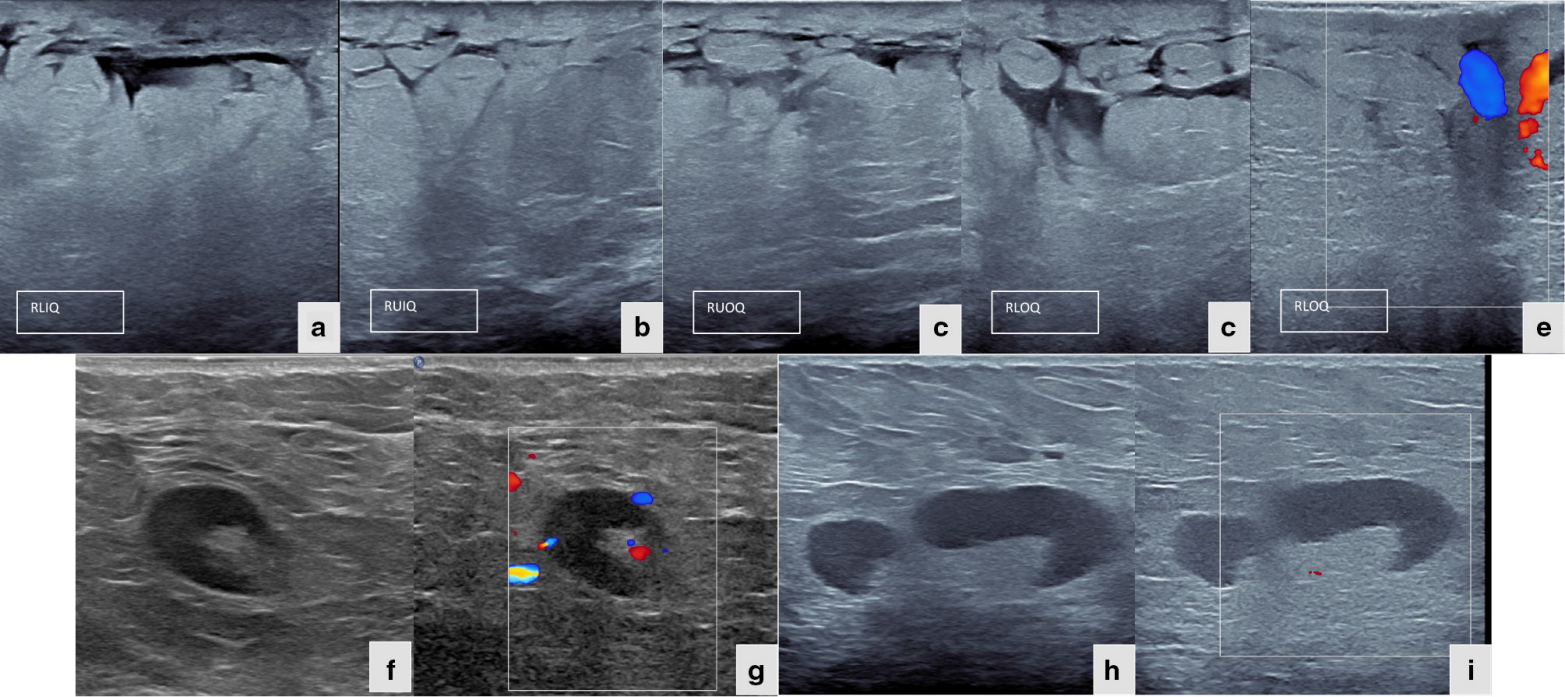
(a–e) Ultrasound and colour doppler of the four quadrants of the right breast shows skin thickening with oedematous changes involving the fat lobules of the breast and mildly increase blood flow. No evidence of ductal dilatation or mass seen. (f–i) Ultrasound and doppler ultrasound of the right axilla shows multiple enlarged lymph nodes with diffuse thicken cortex, however, fatty hilum is preserved with mild increased of blood flow.
